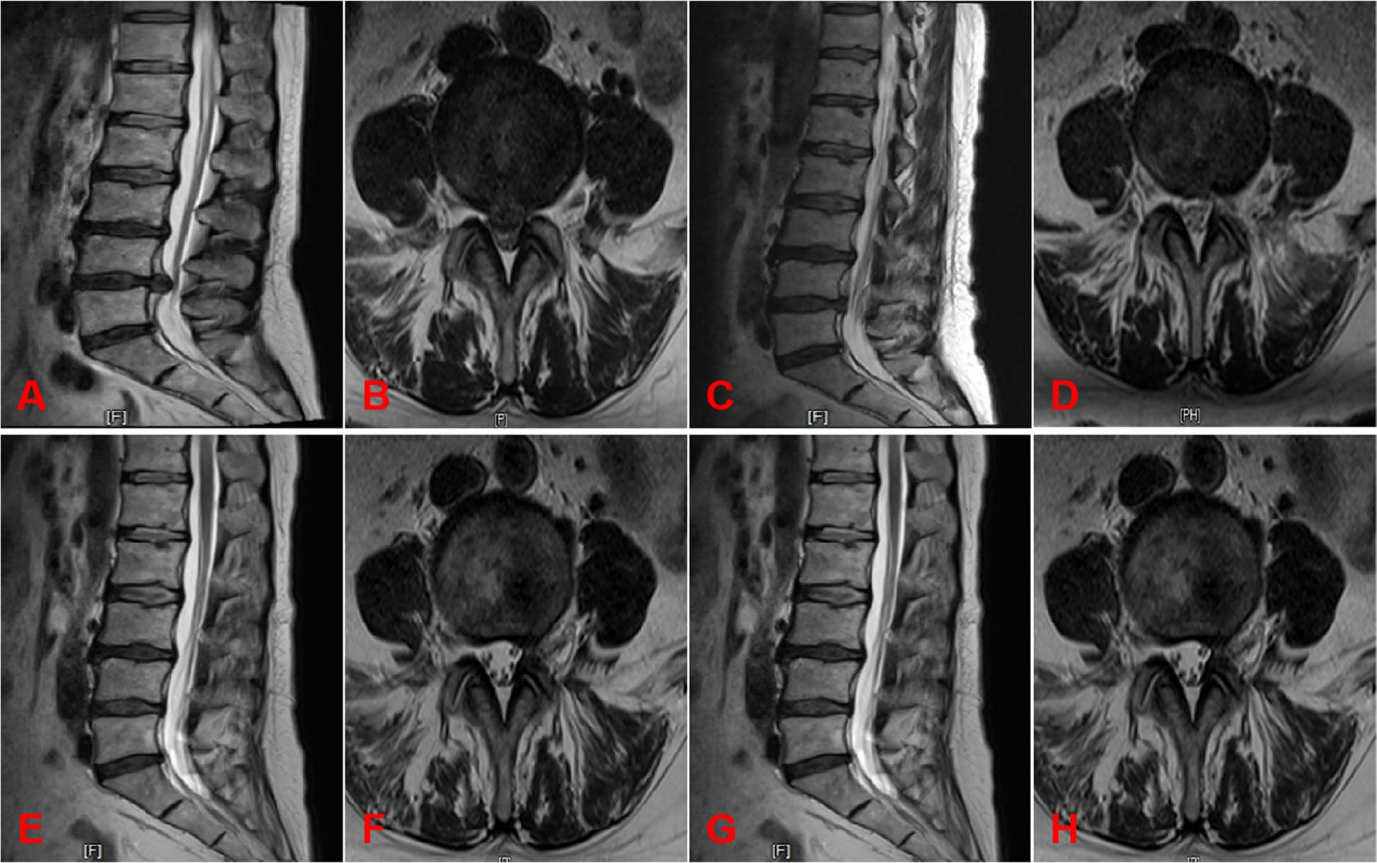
Limited Discectomy. A-B Preoperative sagittal MRI and cross-sectional MRI; C-D sagittal MRI and cross-sectional MRI 3 days after surgery; E-F sagittal MRI and cross-sectional MRI 1 year after surgery;
